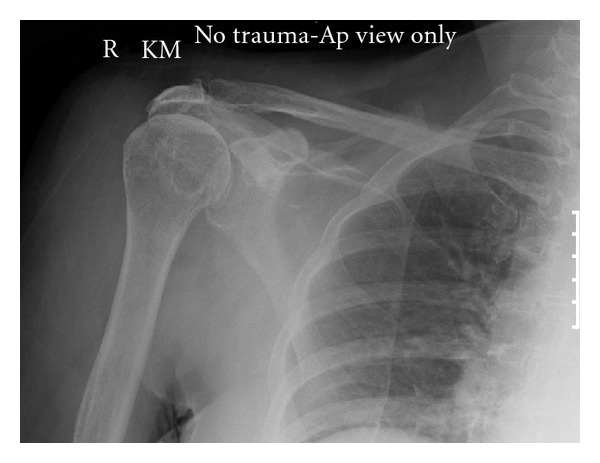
Anterior-posterior radiograph of the right shoulder at admission.
